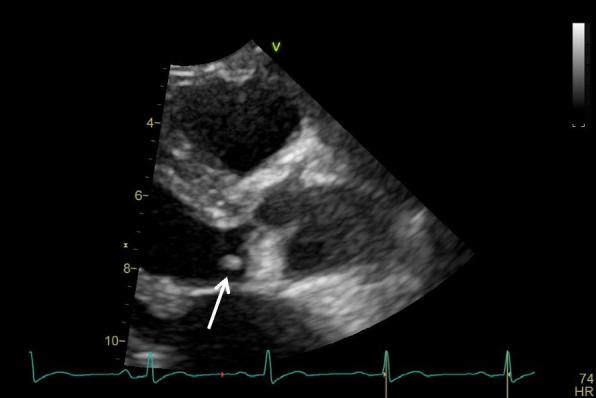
Image of transthoracic echocardiography (TTE). On the TTE, a vegetation (9.0 × 3.0 mm) on the noncoronary cusp of the aortic valve (white arrow) was identified
